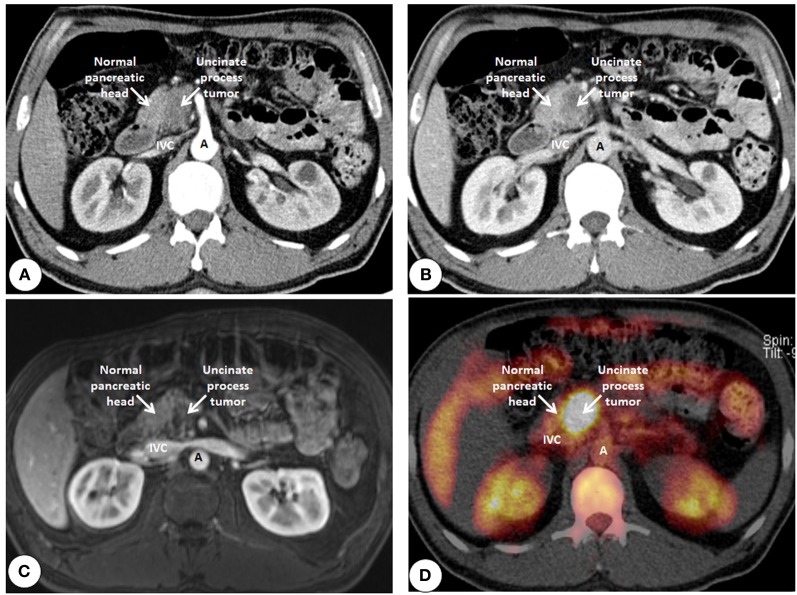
Cross sectional imaging of an uncinate process ductal adenocarcinoma using tools of conventional radiology. (A) Computed tomography using intravenous contrast enhancement (arterial phase) shows the delayed contrast uptake of the fibrotic tumor (darker) compared to the normal pancreas (lighter). (B) Data acquisition during the venous phase shows an increase of contrast difference due to the reduced perfusion of the fibrotic tumor compared to that of the normal pancreas. (C) Contrast enhanced imaging using magnetic resonance imaging showing the tumor also darker than the normal pancreas due to the delay in contrast uptake. (D) Positron emission tomography using radiolabeled glucose (Fluorodeoxyglucose^18^) shows the increased tracer uptake in the tumor compared to that of the normal pancreas. IVC, Inferior vena cava; A, Aorta.
